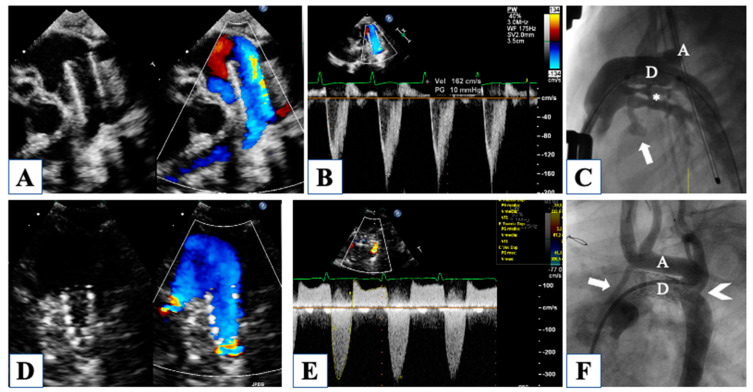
Hybrid stage I palliation. In panel (A), the ductal stent is well expanded, and color Doppler shows normal flow velocity through the stent, as confirmed by pulsed-wave Doppler in Panel (B). In Panel (C), the angiogram delineates clearly the ductal arch (D), the banded pulmonary arteries (*), and the retrograde perfusion of the aortic arch (A) with severely hypoplastic aortic valve (arrow). In panel (D), an example of obstruction at the distal end of the ductal stent, as evidenced by the aliasing (similar for the stent and for the banded pulmonary branch on the left) and by the continuous wave Doppler in Panel (E). Panel (F) shows a potential mechanism of distal obstruction of the stent, with prominent posterior aspect of the aortic isthmus (arrowhead); the arrow is pointing to the severely hypoplastic ascending aorta, while transverse aortic arch has normal diameter (A); ductal stent is well visible (D).
